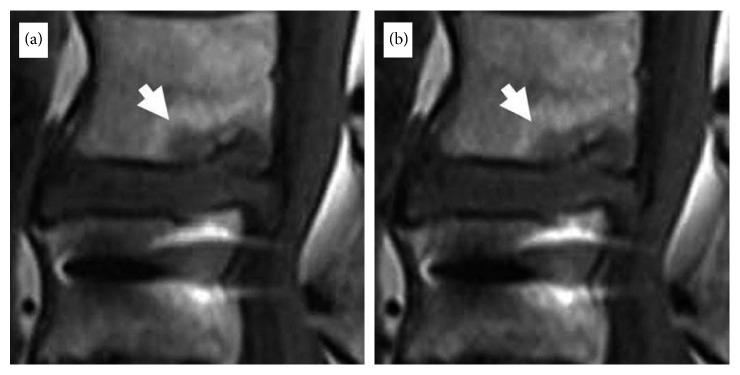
Magnetic resonance imaging (MRI) of a 65-year-old male patient who had fusion surgery previously and was suffering from back pain and buttock pain for more than 28 months. The preoperative VAS score of 7 decreased to 1 postoperatively. (a) Preoperative T1WI lumbar magnetic resonance imaging (MRI) sagittal view with Modic type 1 changes. (b) T1WI lumbar MRI taken after 6 months of the TEBLA sagittal view showing resolution of the Modic reaction (white arrow).
